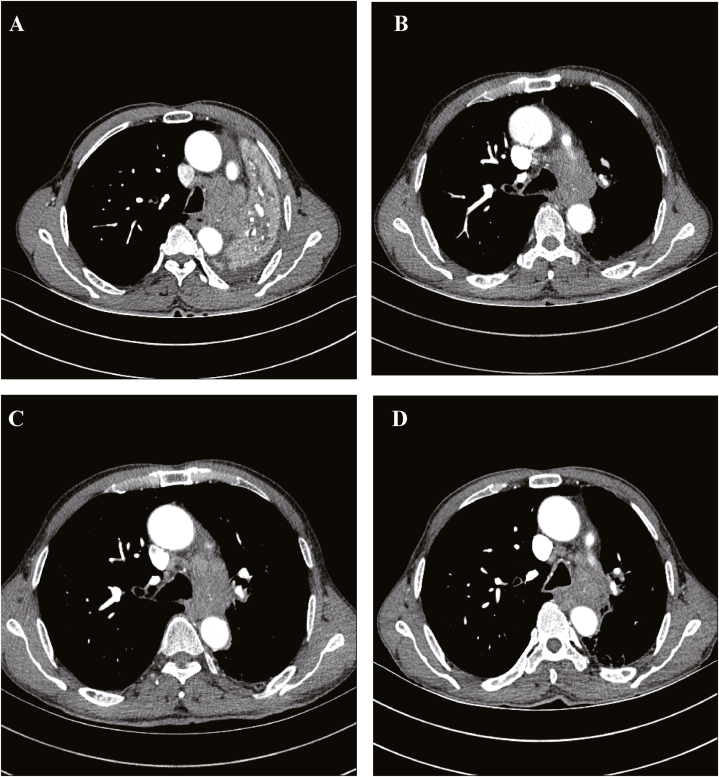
Lung changes on computed tomography (CT) during afatinib treatment. (A) Pretreatment. (B) CT scan at two months after afatinib treatment initiation. (C) CT scan at five months after afatinib treatment initiation. (D) CT scan at eight months after afatinib treatment initiation.
